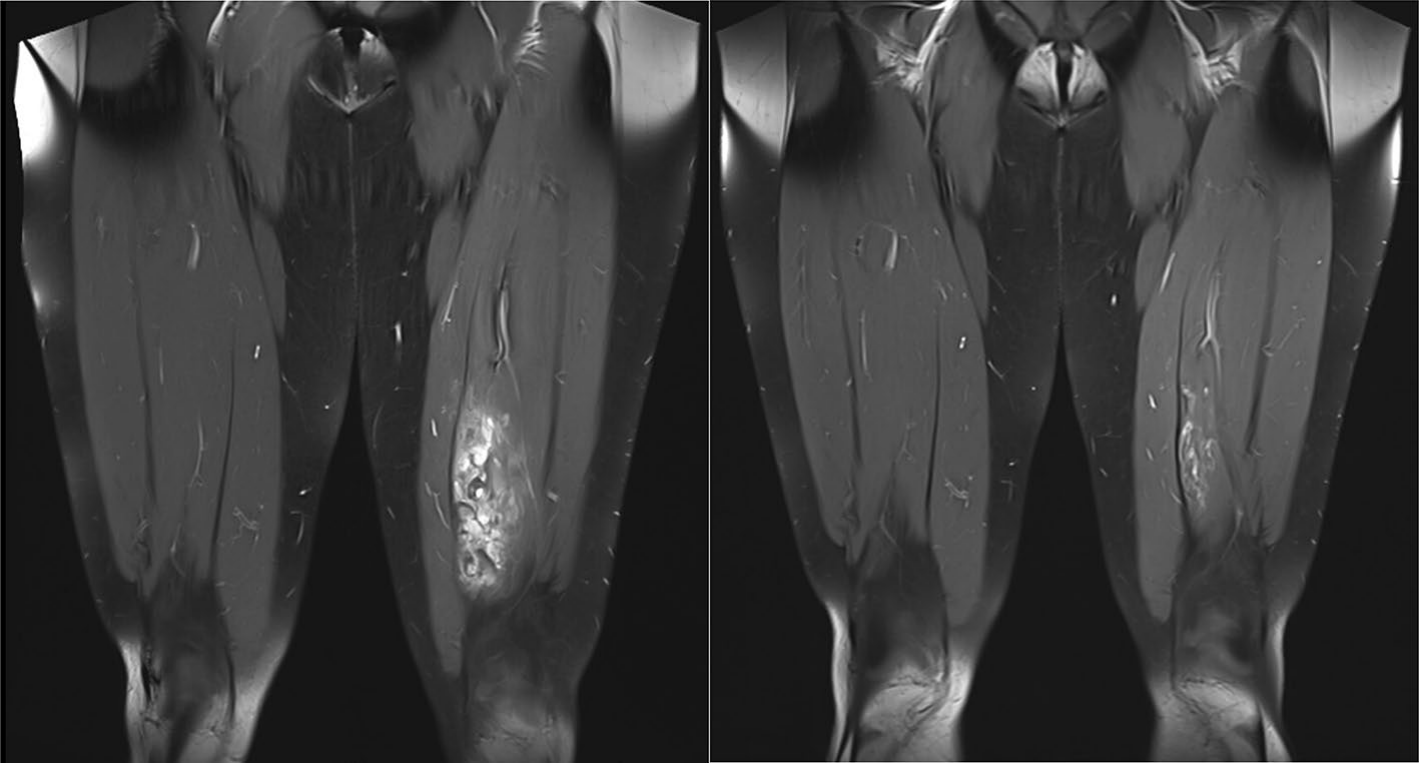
Patient number 12. MRI (T1 STIR cor. Plane) of the thighs prior (left) and 3 months after alpelisib treatment (right).
